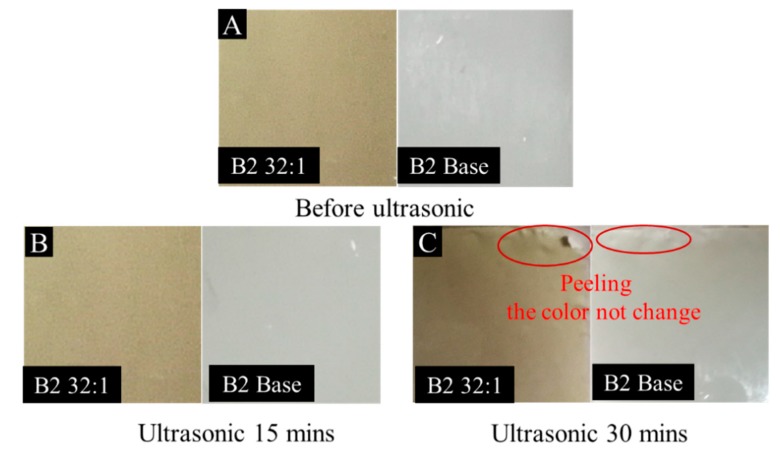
Digital photographs of B2 Base and B2 32:1 membrane before (A) and after ultrasound (B: ultrasound 15 mins; C: ultrasound 30 mins).
